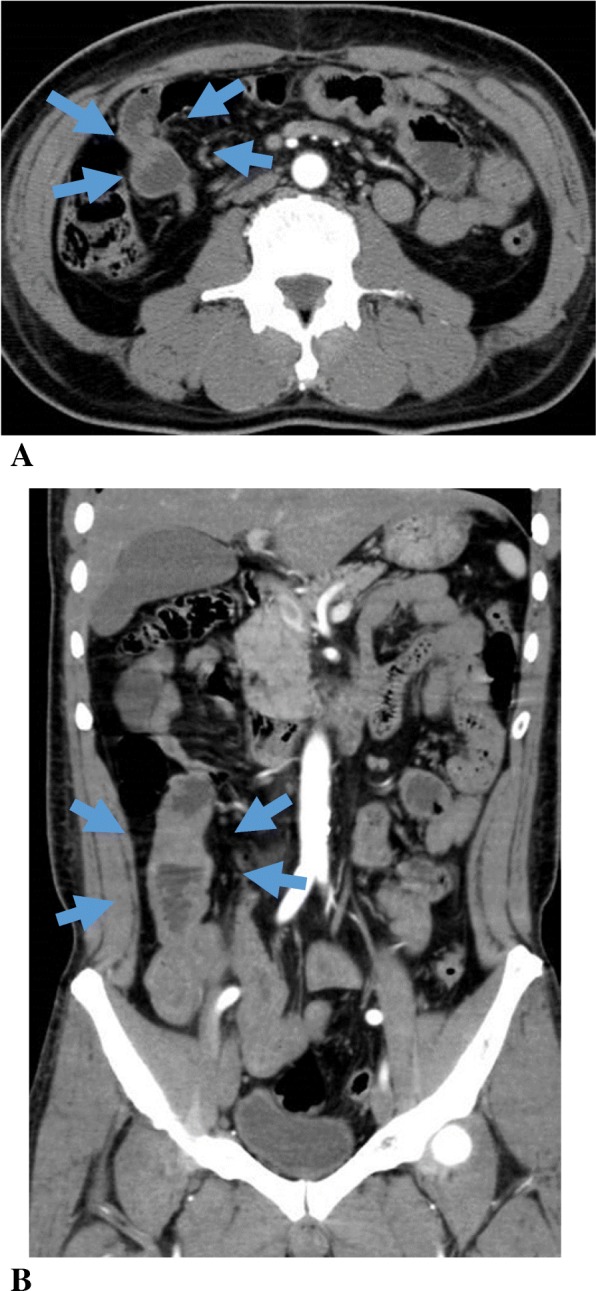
Contrast-enhanced abdominal computed tomography shows thickening of the small bowel wall. a Axial view. b Coronal view
